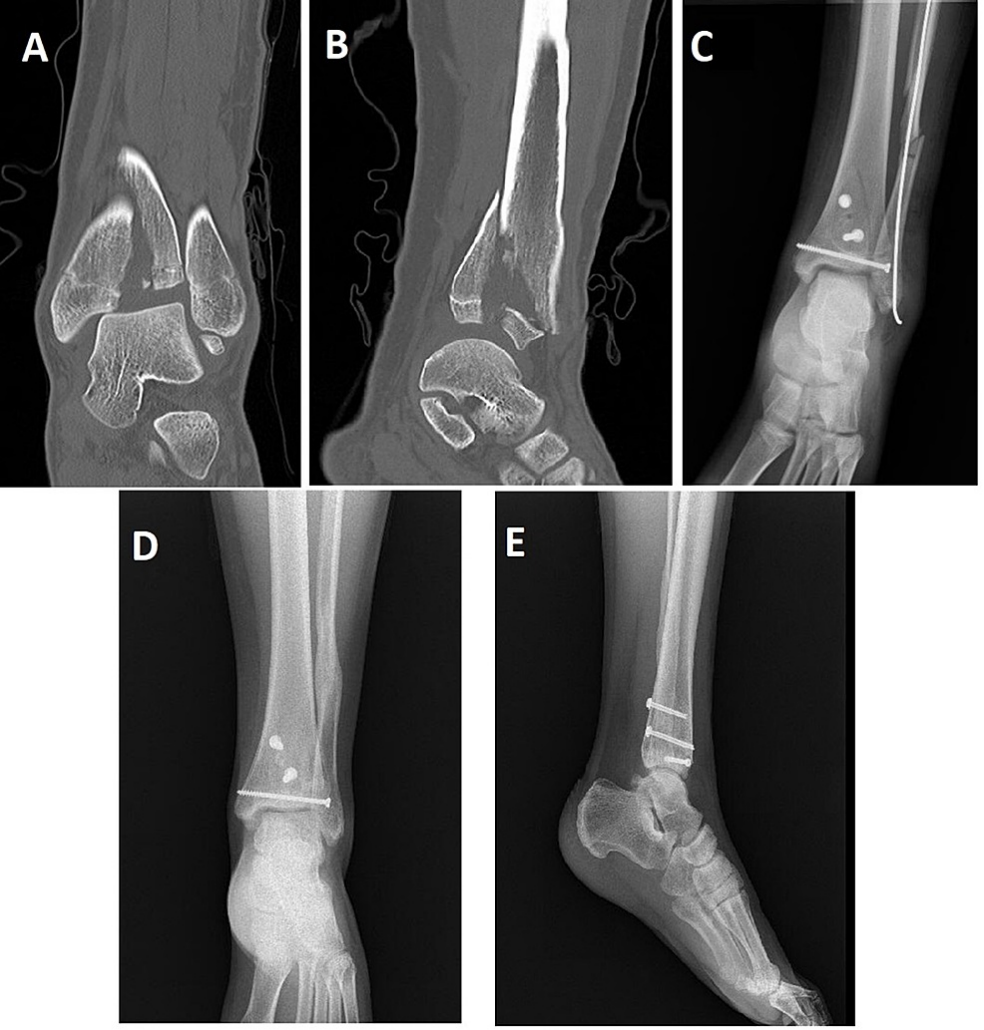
Radiological Evaluation - Preoperative, postoperative, and follow-up imaging of the left ankle triplane fracture A: Preoperative sagittal CT image of the ankle showing the displacement and configuration of the fracture; B: Preoperative coronal CT image of the ankle showing the displacement and configuration of the fracture; C: Anteroposterior radiograph of the ankle showing the postoperative anatomical reduction and fixation, as well as the position of the implants that have never passed through the physics; D: Anteroposterior radiography of the ankle showing the radiological union of the fracture in clinical follow-ups; E: Lateral radiography of the ankle showing the radiological union of the fracture in clinical follow-ups.
